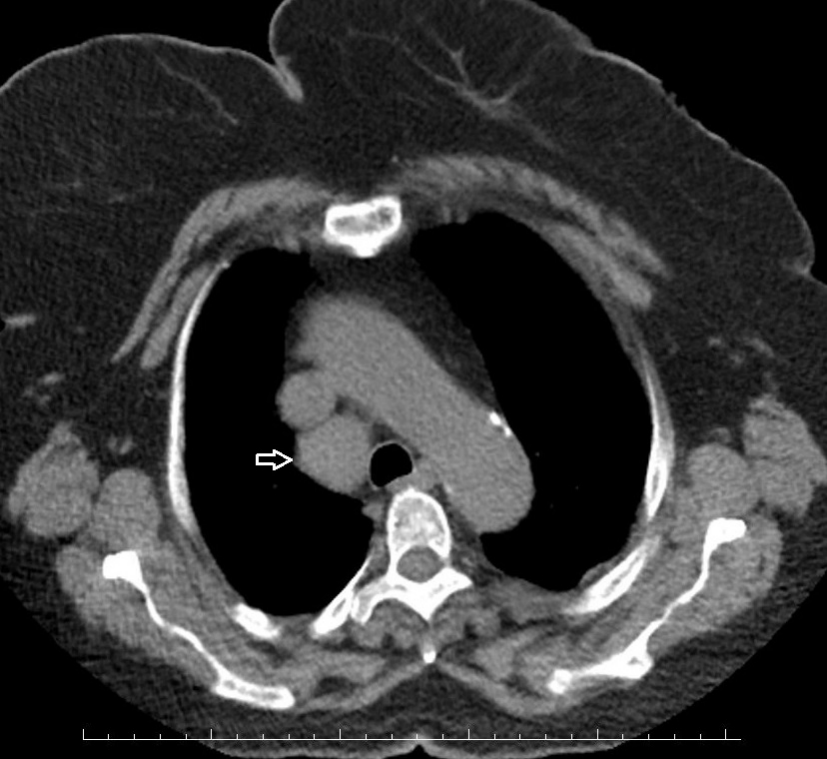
In the chest CT of the patient (64 years age, female, with diabetes mellitus and chronic renal disease), it was seen that there was marked right lower paratracheal (4R) lymphadenopathy in the noncontrast axial section. White arrow: lymphadenopathy.
